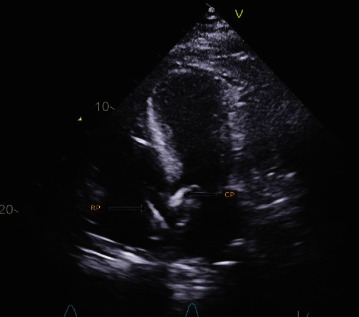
Echocardiographic view of the two pumps in place.
